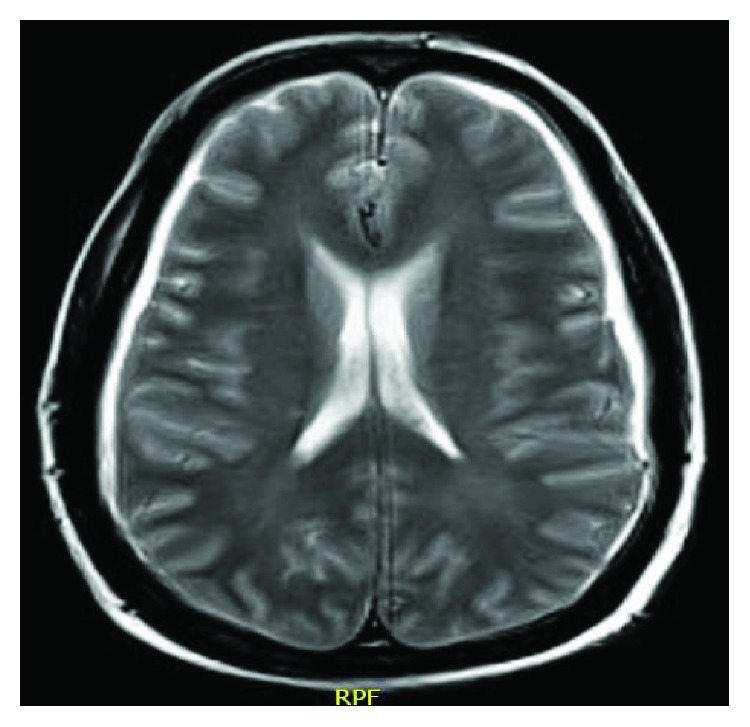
MRI showing leptomeningeal thickening.
